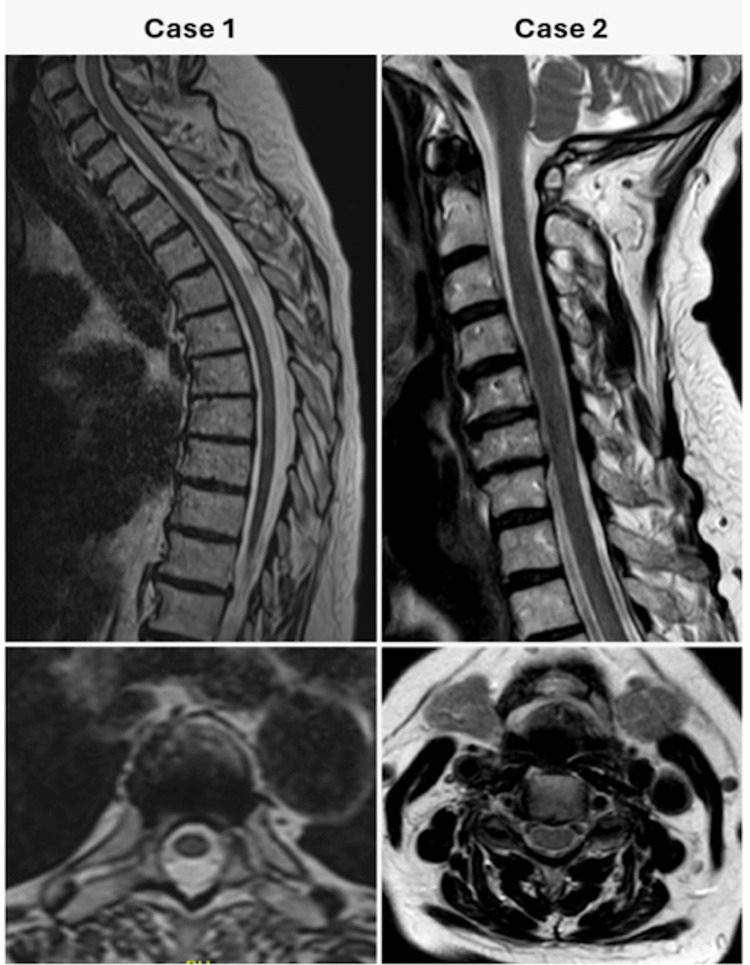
Sagittal (upper) and axial (lower) T2-weighted magnetic resonance imaging (MRI) at follow-up, demonstrating no residual hematoma, spinal cord injury, or underlying lesion.
